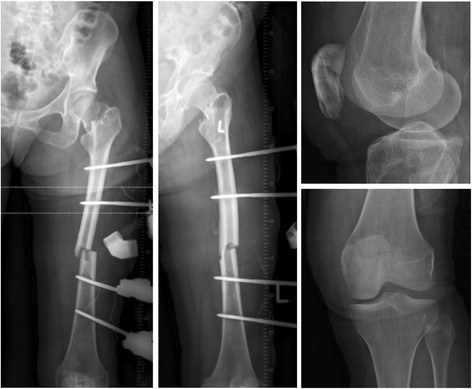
Follow-up radiograph of pelvis at day 1 post-op, demonstrating displacement of the missed left femoral neck fracture and an ipsilateral patella fracture
